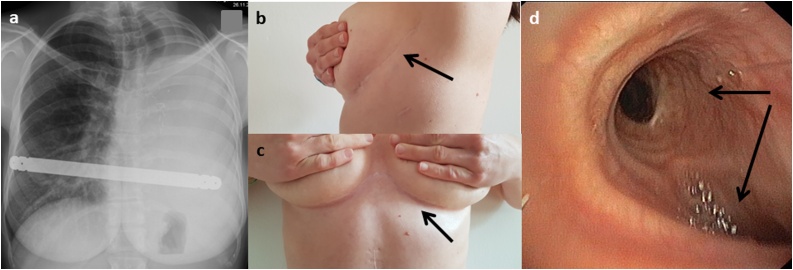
Postoperative results. a) Chest X-ray showing the mediastinum slightly rotated to the left, the pectus bar in correct position. b) Scar of the anterolateral thoracotomy. c) Scar of the submammarian incision to correct the pectus deformity. d) Bronchoscopic views showing open tracheal lumen and no compression of the right main bronchus. Black arrows showing the right main bronchus and the left main bronchus stump.
